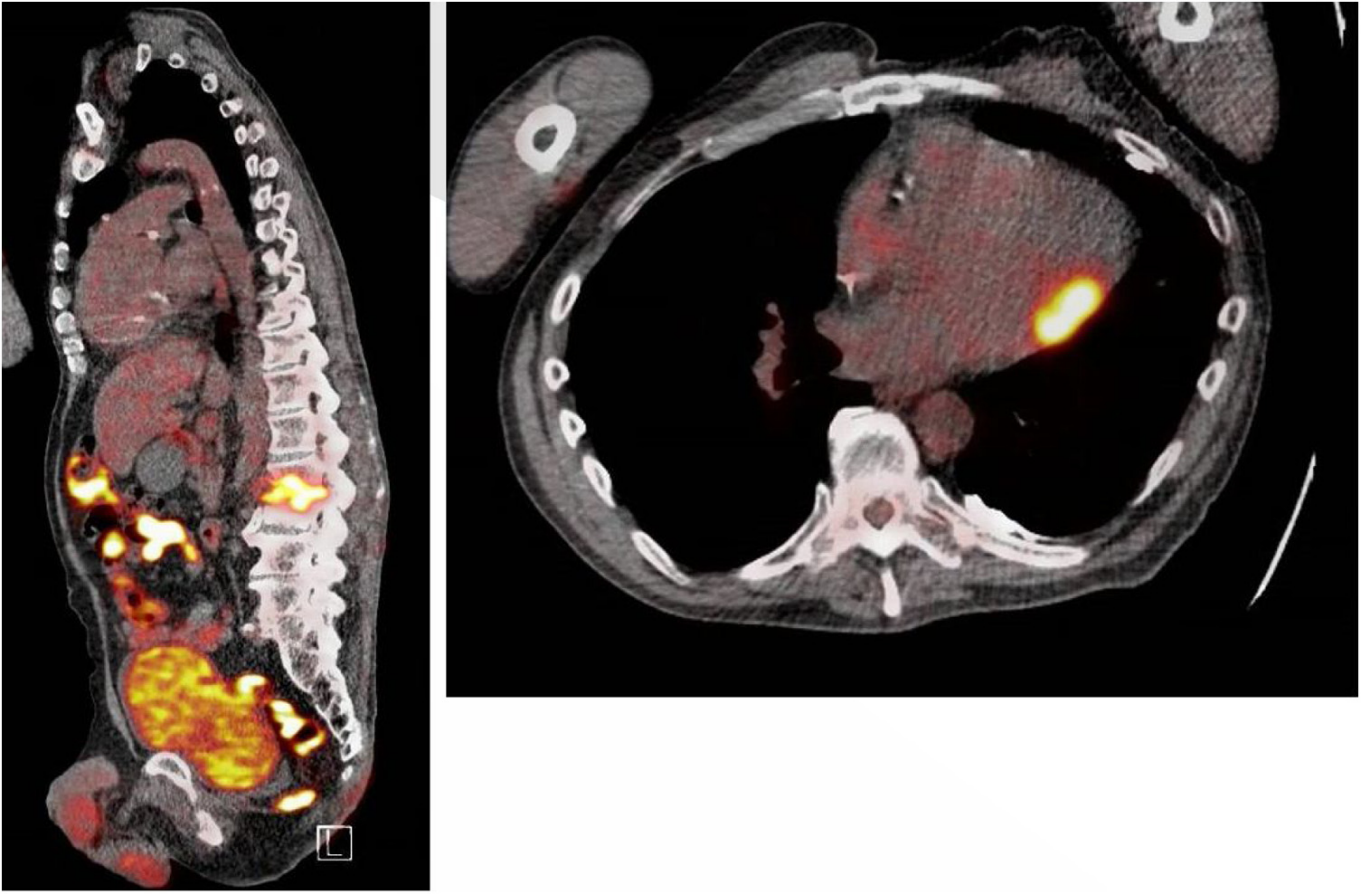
PET-CT scan of patient A showing increased FDG-uptake at lumbar discus L2-L3 (left panel) and in the heart (right panel).
